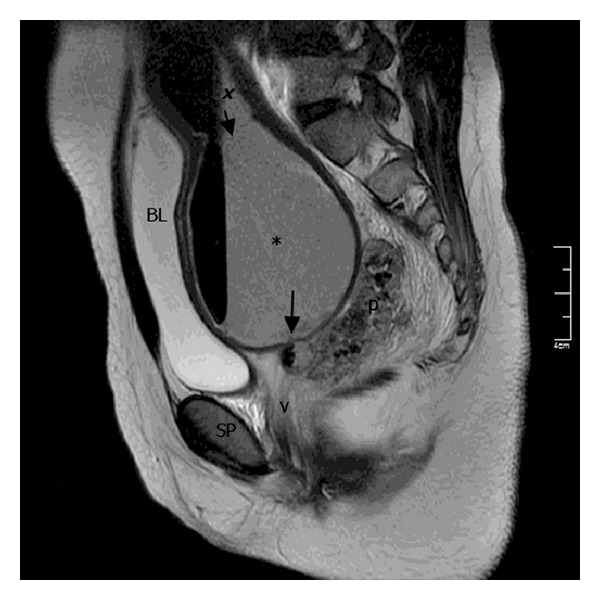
MR imaging demonstrating pus within a distended vagina, endometrial and endocervical canal, and posterior vaginal extraperitoneal leakage (p). A complete mid-transverse vaginal septum was confirmed surgically. Sagittal T2-weighted fast spin echo (FSE) image shows distention of the endometrial (*x*), endocervical (short arrow), and vaginal (*) canals by low-signal-intensity material with air-fluid level representing pus. Long arrow is pointing at a fistula tract (a collection) between the vagina and rectum. The level of the obstruction lies above the symphysis pubis (SP).
